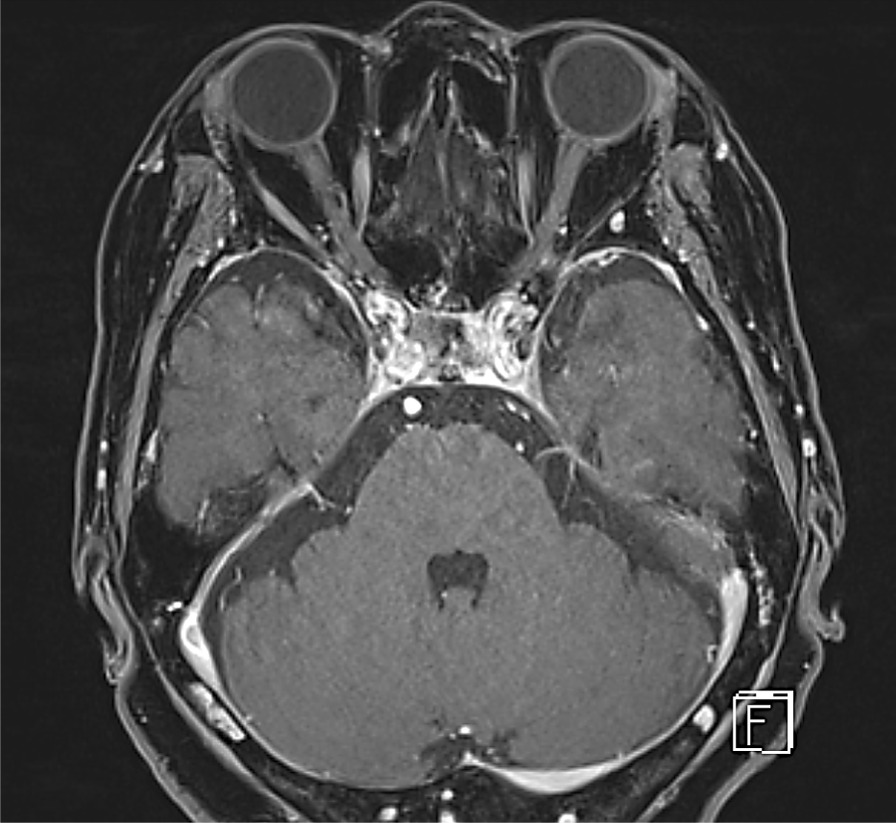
Gadolinium-enhanced T1-weighted MRI image showing no abnormal mass lesions or enhancement within the cavernous sinus, axial view
